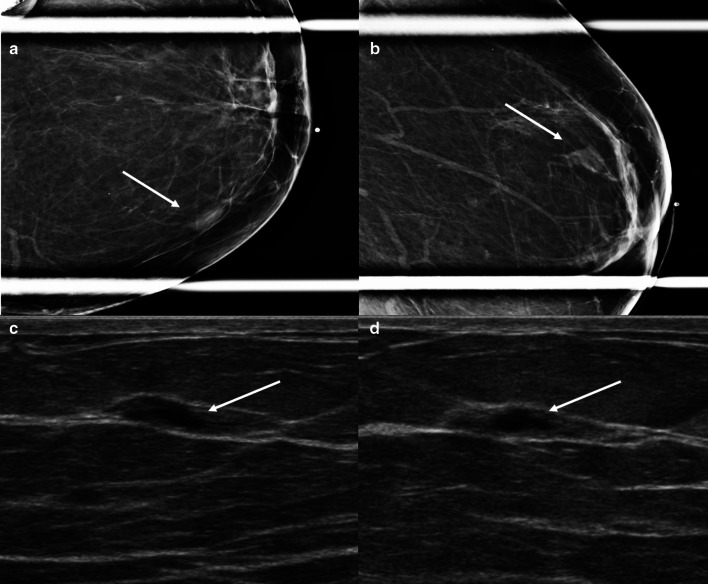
Stromal fibrosis. A 55-year-old woman presented for a focal asymmetry detected on screening mammography. Craniocaudal (a) and lateral (b) spot compression mammographic views show an oval non-calcified mass with angular margins (arrows). Grayscale transverse (c) and longitudinal (d) ultrasound show a mixed-echogenicity oval mass correlating with the mammographic finding (arrows). Ultrasound-guided biopsy yielded stromal fibrosis. Six-month follow-up mammography and ultrasound were recommended and demonstrated stability
